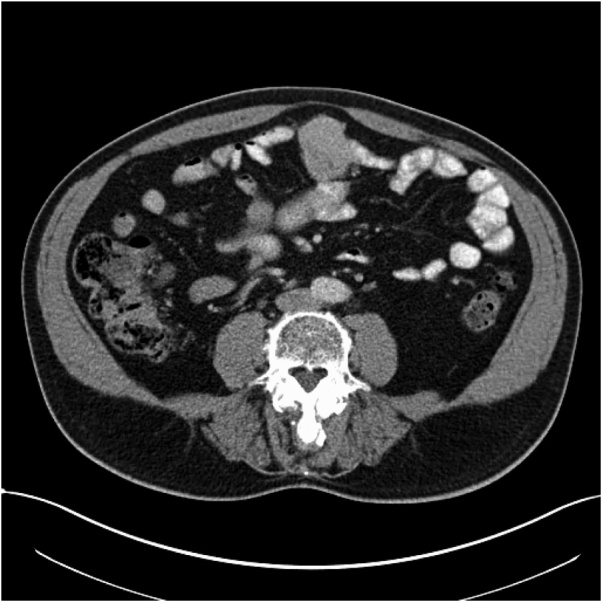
Coronal view of CT AP showing 4.5 cm lobulated mass in the anterior abdominal wall.
